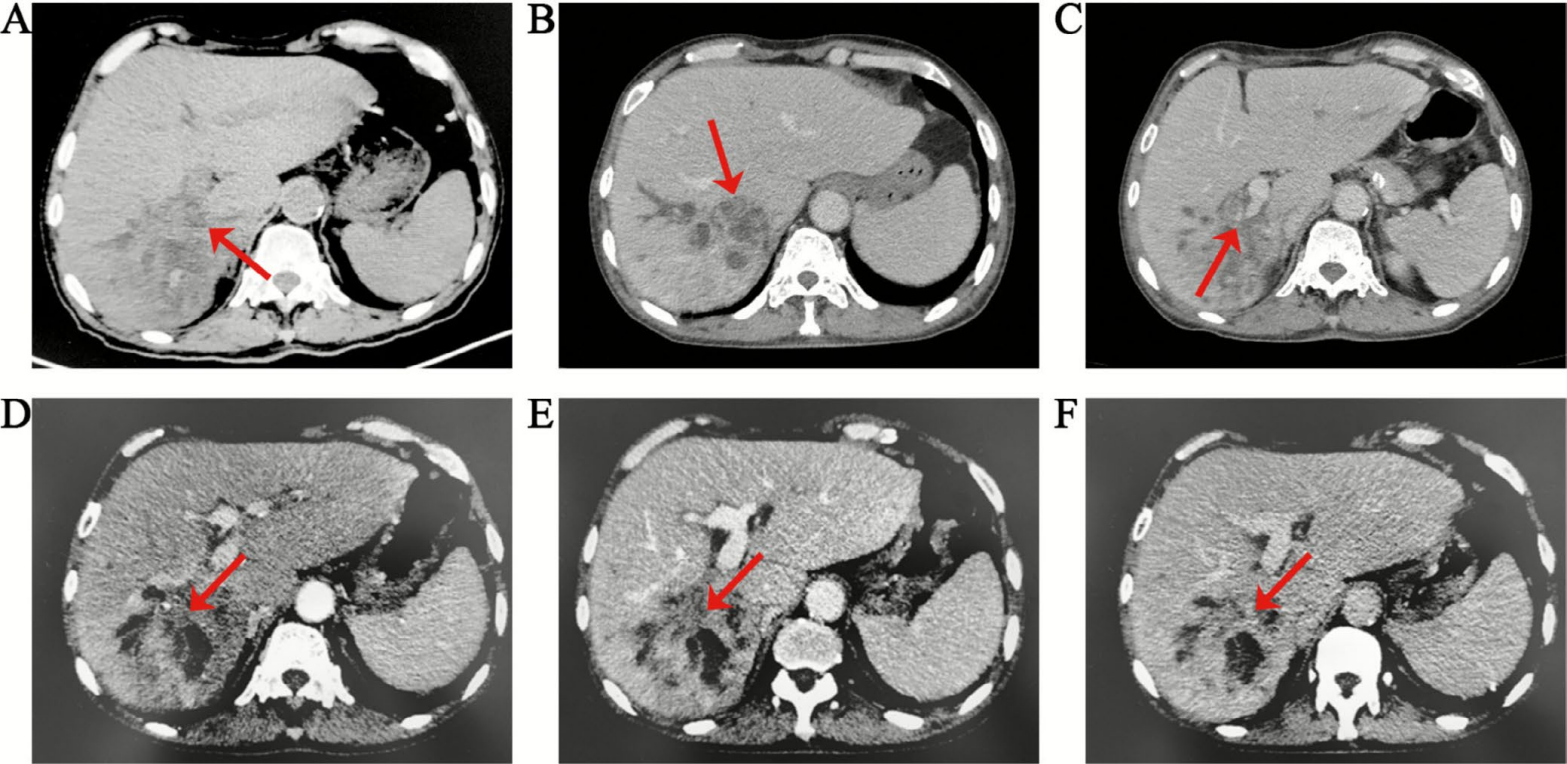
Computed tomography (CT) and contrast enhanced CT. (A) On plain CT scan, the density of liver parenchyma was slightly reduced; (B) Enhanced CT showed a honeycomb‐like low‐density shadow in the right sector (segments V to VIII) near the hepatic hilum; (C) Enhanced CT showed that the intrahepatic segment of the right portal vein was nearly occluded; (D–F) Enhanced CT showed thickening of the intrahepatic bile duct wall in the right lobe near the hepatic hilum with mild delayed enhancement (D: Arterial phase, E: Venous phase, F: Delayed phase).
